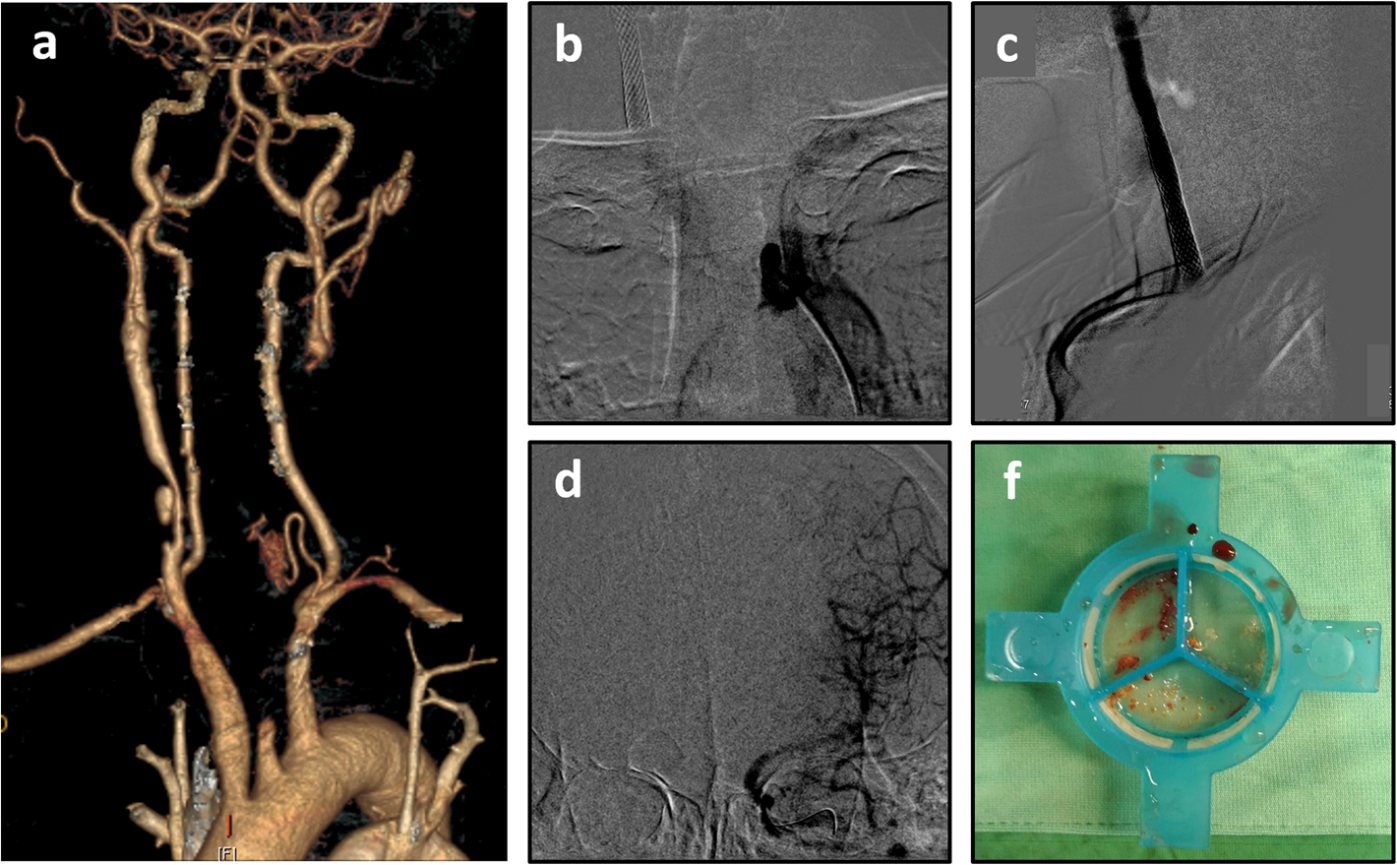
Patient 2 computed tomography angiography (a). Angiography (b). Final angiography (c). Intracranial angiography after the intervention (d). Thrombus (e)
